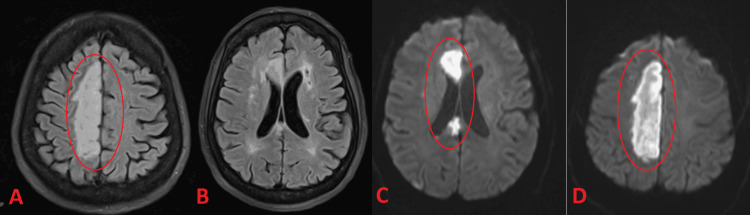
MRI confirming acute bilateral anterior cerebral artery (ACA) infarction A and B (FLAIR): A hyperintense signal is observed along the medial (parasagittal) surfaces of both frontal lobes, indicating acute infarcts in the ACA territory. Mild periventricular hyperintensities reflect chronic small‐vessel ischemic changes. C and D (DWI): A bright (restricted) signal in the same medial frontal regions confirms acute infarction bilaterally; the small‐vessel changes remain non‐restricted and chronic. FLAIR: fluid-attenuated inversion recovery; DWI: diffusion-weighted imaging
